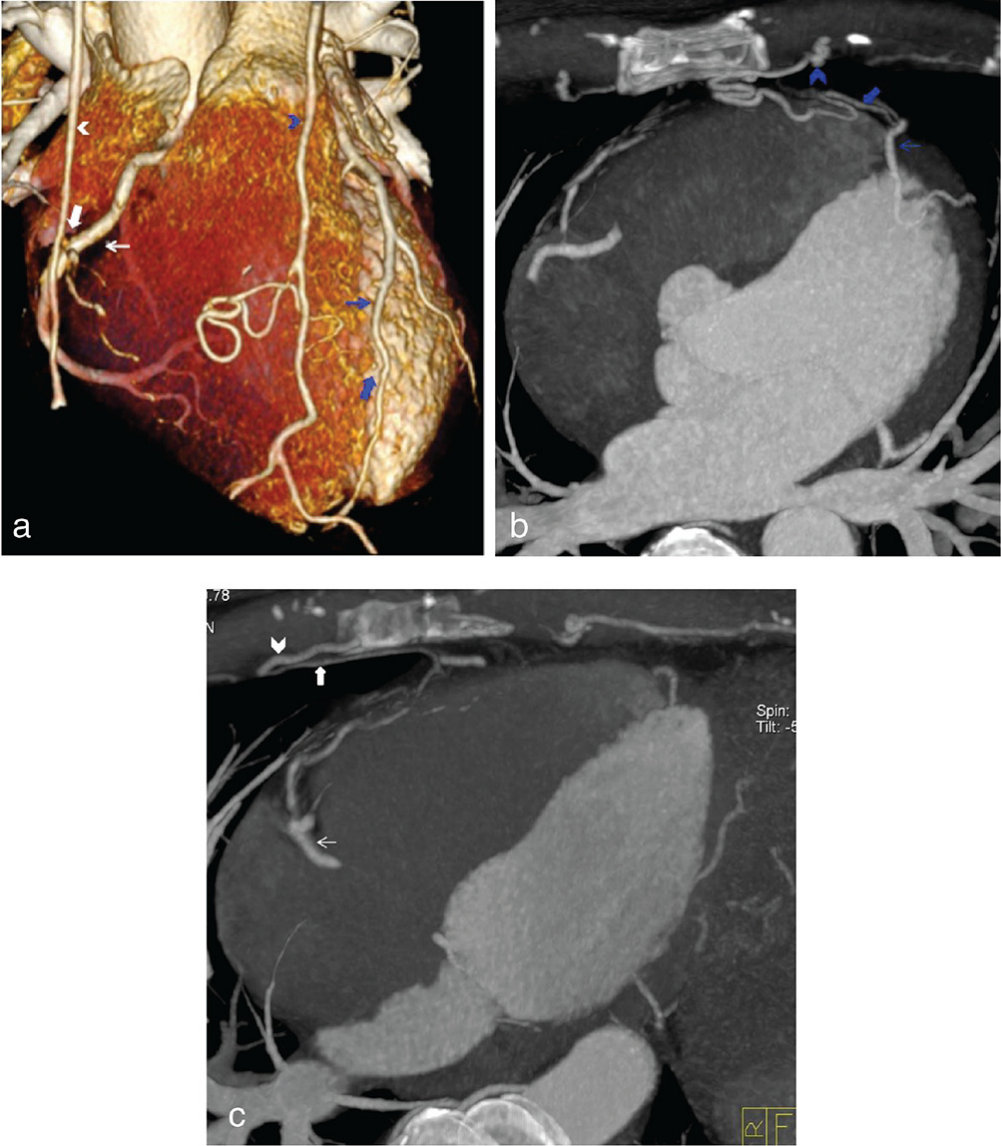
(a) Volume-rendered cardiac CT image demonstrates the fistulous communication (white and blue block arrows) between the right internal mammary artery (white chevron) and mid-right coronary artery (thin white arrow), and left internal mammary artery (thin blue chevron) and mid left anterior descending artery (thin blue arrow). (b, c) CT axial curved maximum intensity projections demonstrating the fistulous communication (white and blue block arrows) between the right internal mammary artery (white chevron) and mid-right coronary artery (thin white arrow), and left internal mammary artery (thin blue chevron) and mid left anterior descending artery (thin blue arrow).
